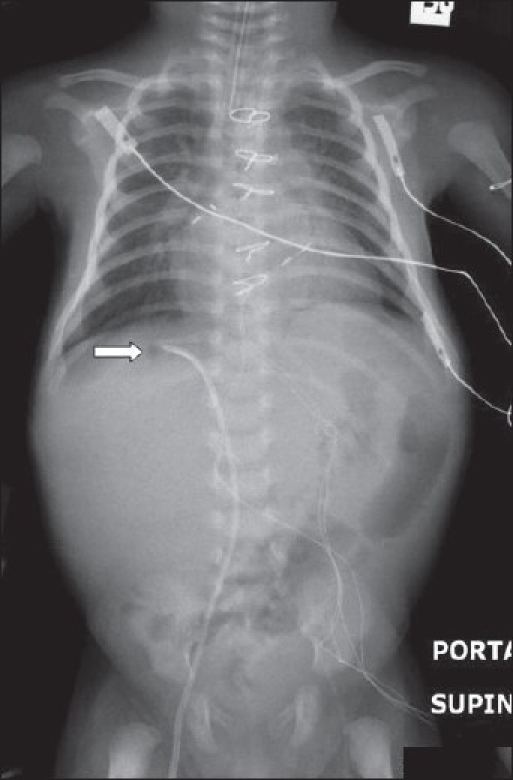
Radiograph of chest and abdomen of a neonate following cardiac surgery, showing a misplaced intravenous line into a hepatic vein. The intravenous line has been introduced via the right femoral vein
